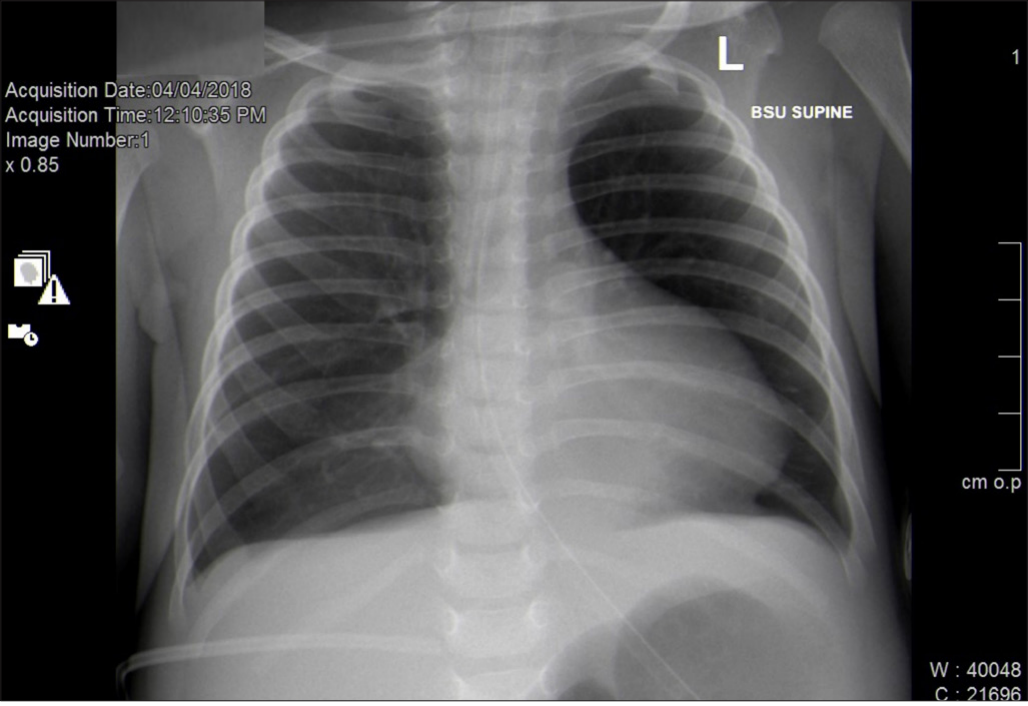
Chest X-ray, showing poor visualisation of the airway and hyperinflation.
